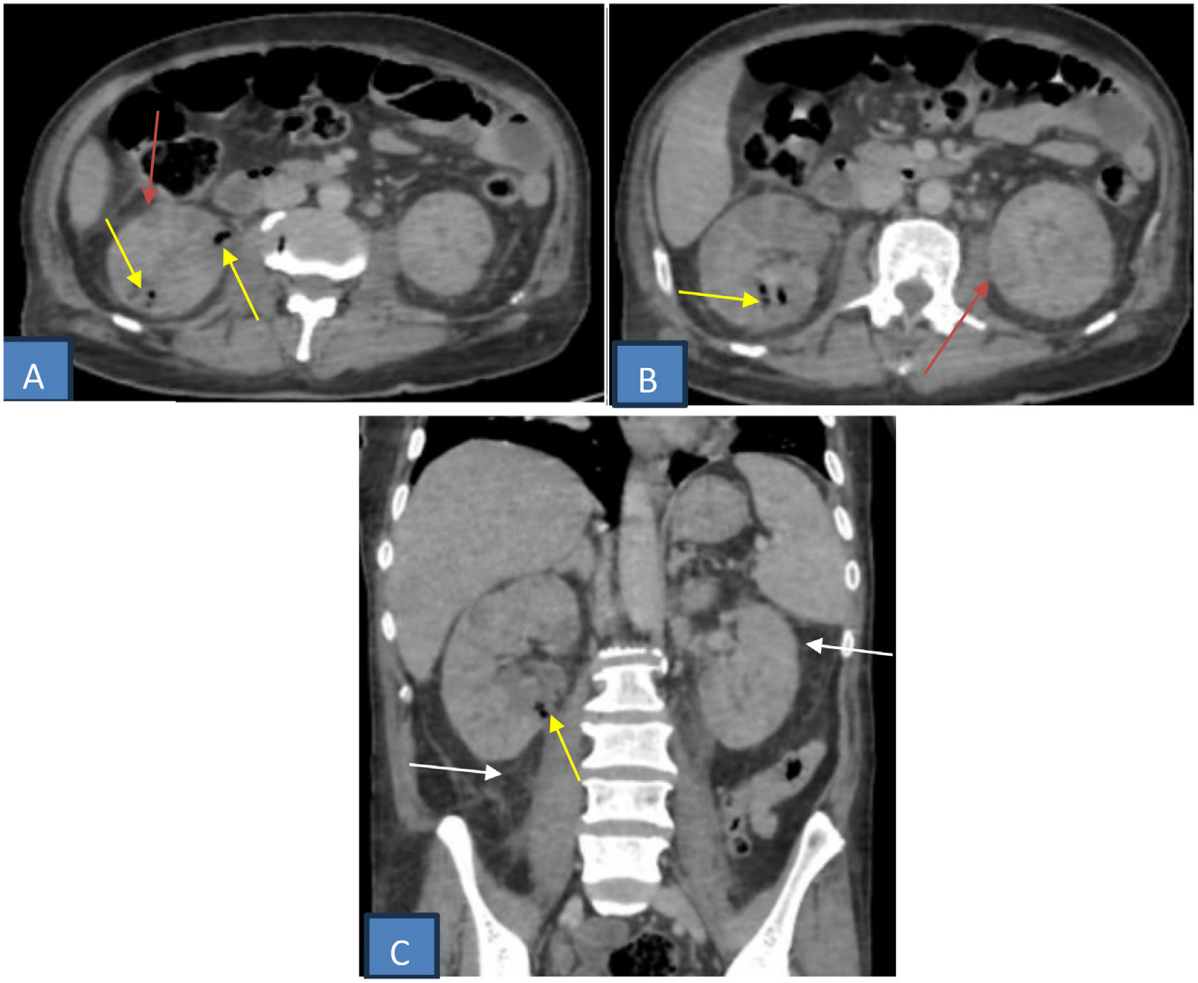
Axial (A and B) and coronal (C) contrast-enhanced CT scan showing an enlarged right kidney with multiple gas locules in the calyceal system (yellow arrow), consistent with emphysematous pyelonephritis. Bilateral renal parenchymal hypoenhancement (red arrow) and perirenal fat stranding (white arrow).
